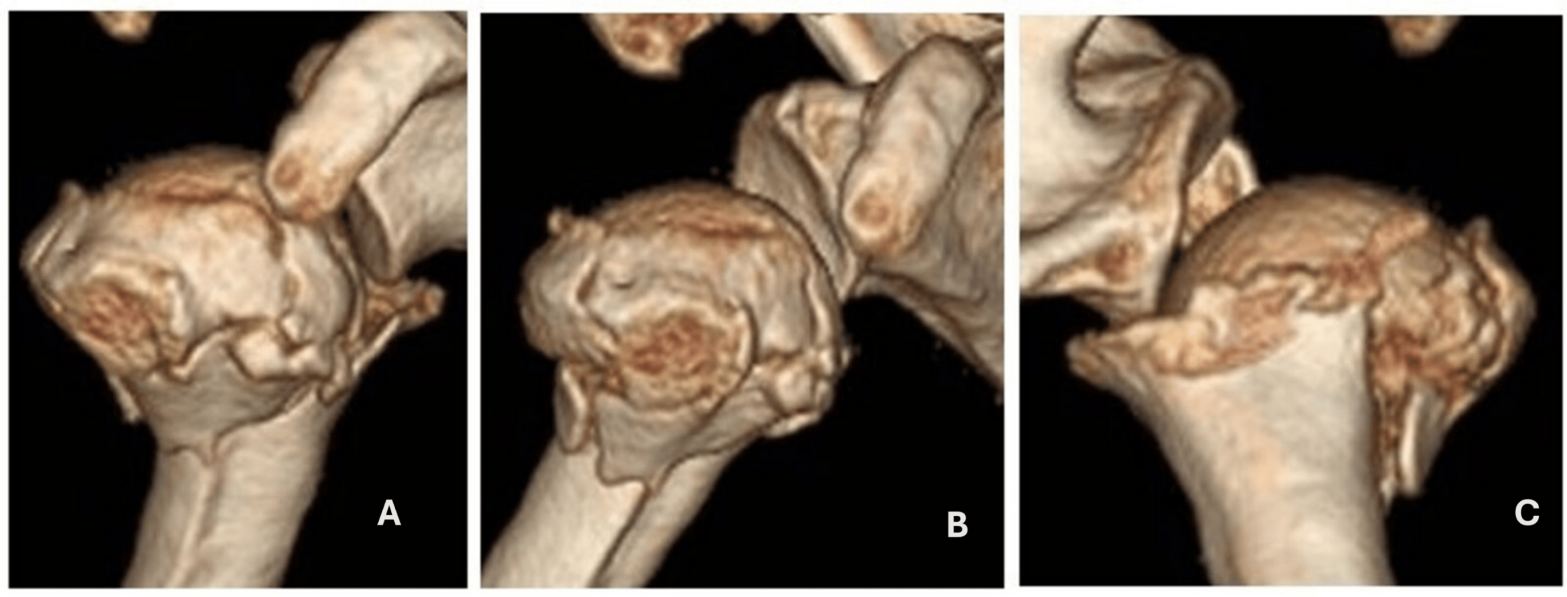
Computed tomography in 3D reconstruction with anterior view (A), anterolateral view (B), and posterior view (C), providing a better understanding of the fracture line.
